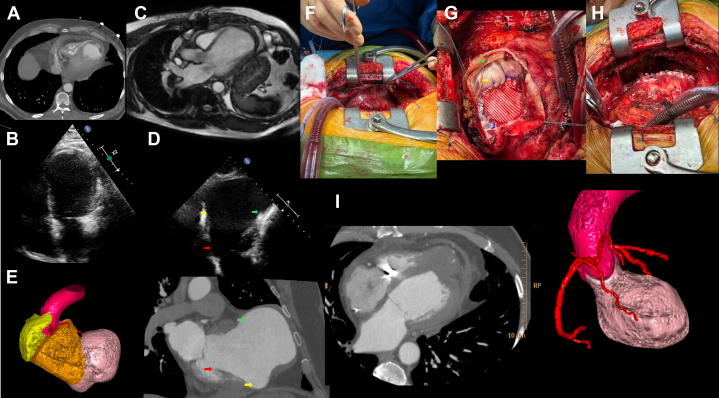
Case Presentation and Surgical Management (A) 2021 CT scan with thinning of the apical left ventricular wall with contained myocardial rupture subjective of pseudoaneurysm. (B) 2021 TTE with contrast showing aneurysmal dilatation of the left ventricle with apical thinning, absence of pericardial effusion. (C) 2021 MRI showing a dilated left ventricle with marked thinning of the anterior and anteroseptal walls, an apical aneurysm, and mural thrombus in the anterior wall. (D) 2024 TTE showing apical aneurysm and left ventricular enlargement. Shown is the transition zone between true left ventricle cavity (red arrow), aneurysm (yellow arrow) and pseudoaneurysm (green arrow). (E) 2024 CT 3-dimensional reconstruction shows the difference between normal left ventricle and pseudoaneurysm. CT scan to the right shows apical aneurysm and left ventricular enlargement. Transition zone between true left ventricle cavity (red arrow), aneurysm (yellow arrow) and pseudoaneurysm (green arrow). (F) Left ventricular aneurysm adhered to the thoracic wall. (G) Transition zone between true left ventricle cavity below Dacron patch, aneurysm (yellow arrow) and pseudoaneurysm (green arrow). (H) Left ventricle after repair. (I) Postoperative CT scan shows a nondilated left ventricle, absence of the previously seen pseudoaneurysm, presence of a Dacron patch at the neo-apex, and no pericardial effusion. CT = computed tomography; MRI = magnetic resonance imaging; TTE = transthoracic echocardiography.•2021 magnetic resonance imaging (MRI): LV dilation (end-diastolic volume: 245 mL, EF: 30%), transmural scar in anterior/anterior-septal walls (Figure 1C).•2022-2024 annual TTE and chest x-ray: gradual enlargement of aneurysm to 88 × 72 mm (Figures 1B and 1C).•2025 CT: the aneurysmal neck measured 68 mm, with a maximal diameter of 98 mm. A suspected pseudoaneurysmal cavity was identified at the inferior margin of the aneurysm, measuring 40 × 20 mm. The indexed LV end-diastolic volume was 482 mL including the aneurysmal component and 285 mL when excluded, confirming the extent of geometric distortion (Figure 1E).•2025 MRI: EF of 28%, large scar burden, confirmed pseudoaneurysm.
